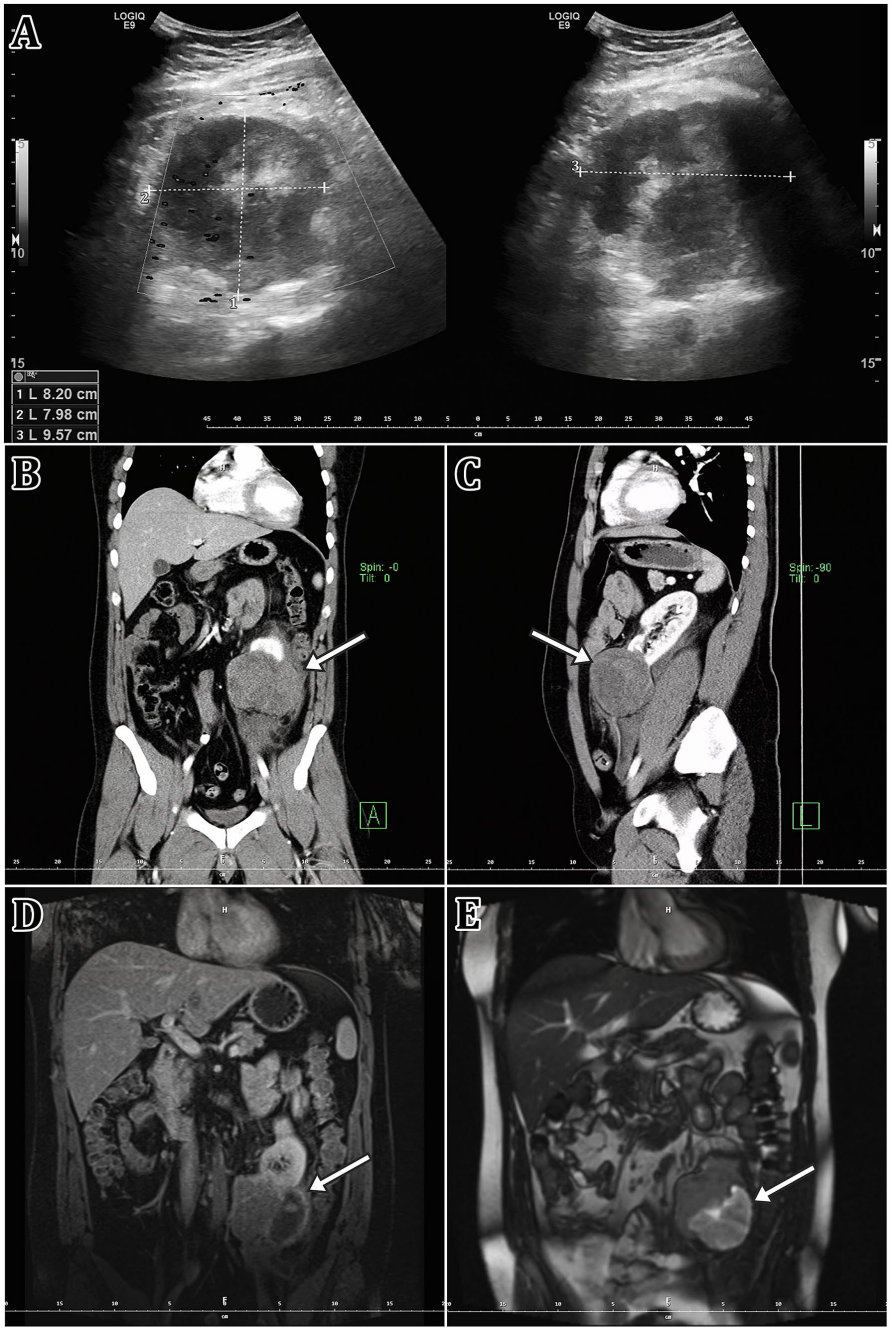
This figure summarises the findings of the primary tumorous focus’s imaging modalities. (A) Ultrasound imaging, (B,C) Computed tomography imaging (CT; contrast enhanced), (D,E) magnetic resonance imaging [MRI; (D) T1, (E) T2 sequence]. A white arrow indicates the tumorous lesion itself.
